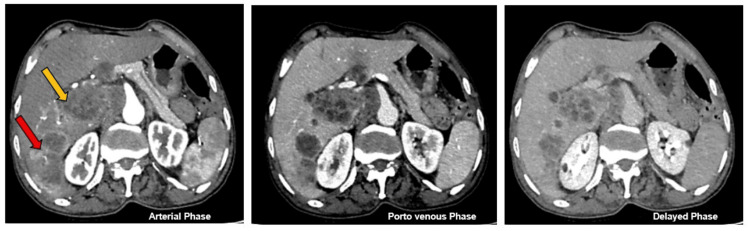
Computed tomography images of the sarcomatoid hepatocellular carcinoma. The red arrow highlights an infiltrative mass in segment VI of the liver, demonstrating heterogeneous peripheral enhancement during the arterial phase with corresponding washout on the portovenous and delayed phases. The yellow arrow indicates a large nodal conglomerate at the porta hepatis exhibiting similar enhancement features.
